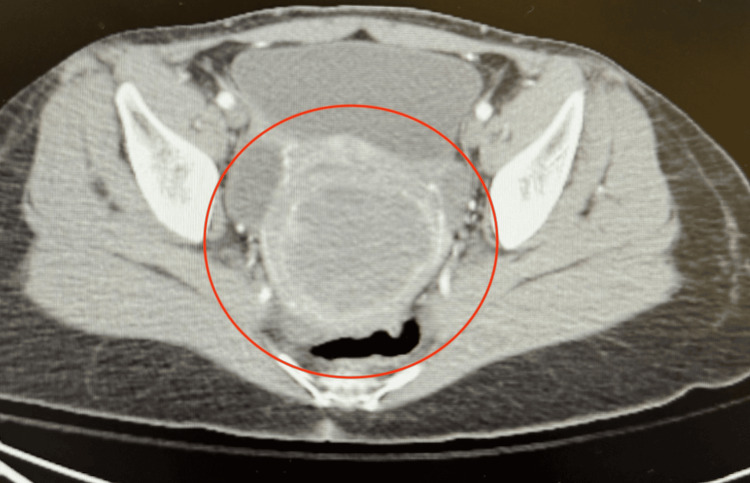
CT of the pelvis revealing a uterine lesion suggestive of a fibroid or cystic change. CT: computed tomography
